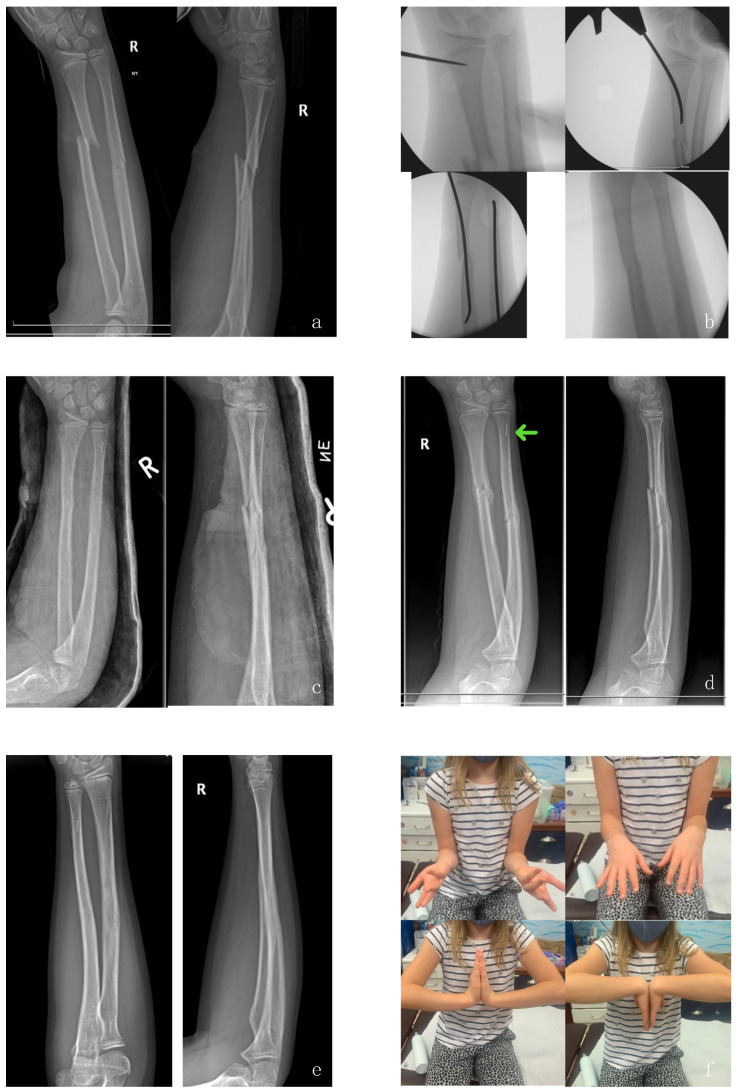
(a) Preoperative X-ray; (b) intraoperative; (c) one week; (d) six weeks (arrow marks the radiopaque tip of the implant); (e) one year; (f) range of motion after one year.
